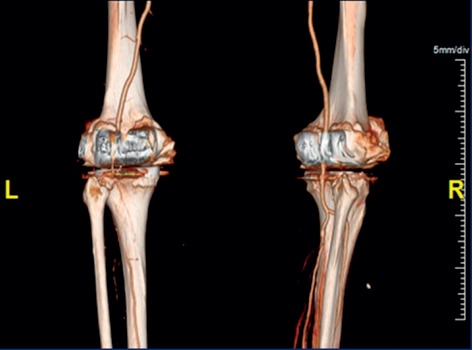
Computed tomography (CT) angiography. This CT image revealed left popliteal, left deep femoral, and left anterior tibial artery occlusion.
